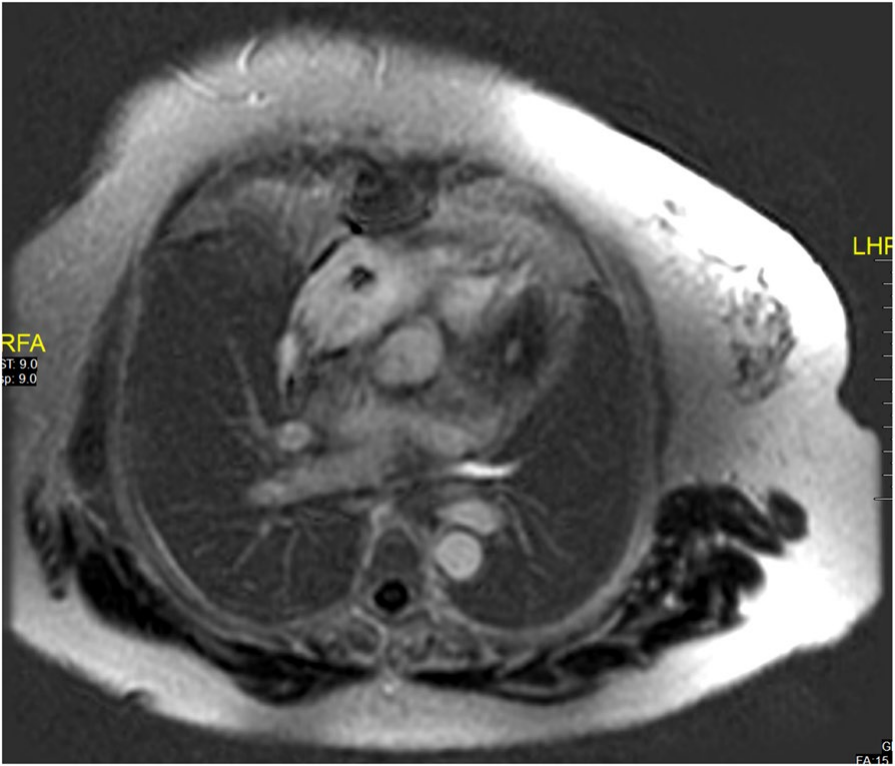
Cardiac magnetic resonance imaging showing subtle residual minimal soft tissue thickening in the middle mediastinum along the pericardium and right atrial walls abutting the SVC with no avidity
